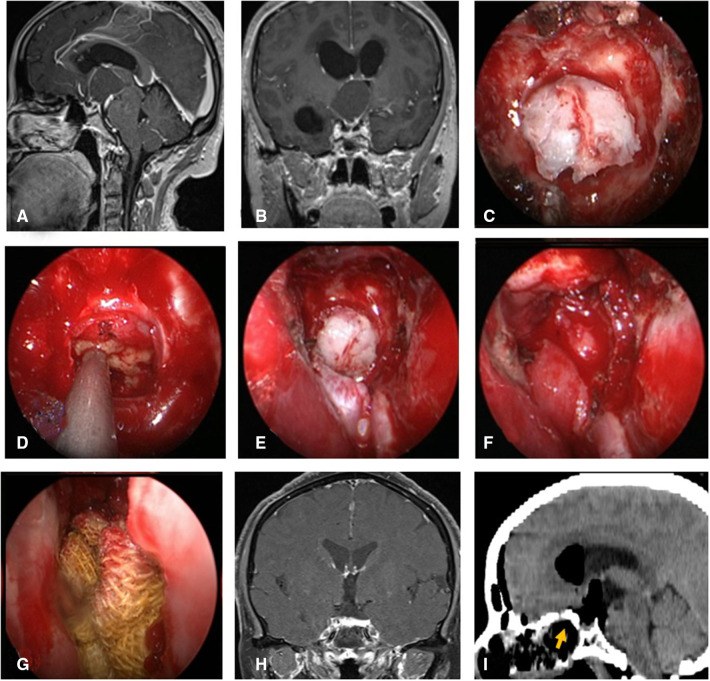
Changes in reconstruction strategy/(after) [(C) ISBF harvesting; (D–G) process of reconstruction]. (A,B) MRI enhanced in sellar region before operation, and craniopharyngioma was considered; .C) Osteoclastic craniectomy to creating a bone flap *in situ*. (D) Subdural fat packing. (E) Repair of skull base bone window with ISBF. (F) Cover the VP-NSF on the ISBF. (G) Gauze support. (H) Coronal enhanced MRI in sellar after operation and postoperative pathology showed craniopharyngioma. (I) CT bone window showing ISBF was in place. VP-NSF, vascularized pedicle nasoseptal flap, ISBF, *in situ* bone flap.
